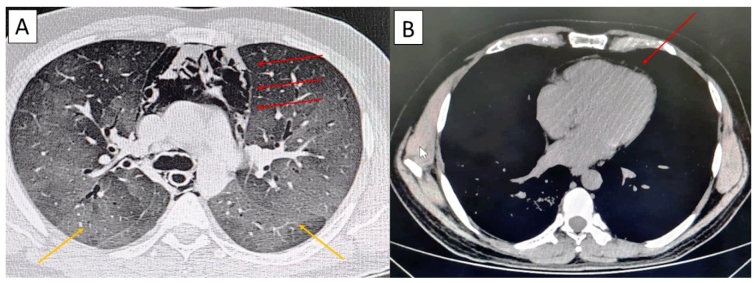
Axial unenhanced chest computed tomography. (A) Large pneumomediastinum (red arrows) and multiple ground-glass opacities (yellow arrows) in both lung fields. (B) Resorption of the pneumomediastinum with a small layer of air (red arrow).
